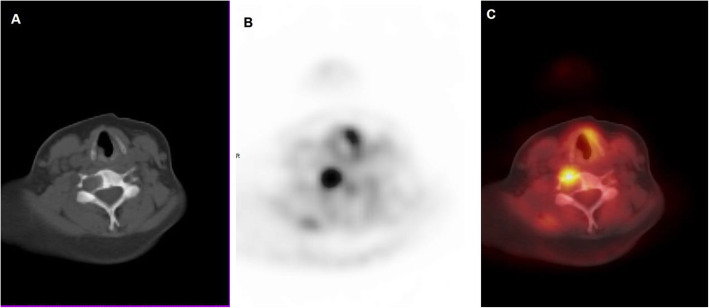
^18^F-FDG PET/CT: A axial computed tomography image showed a lytic lesion in the right side of C6 vertebral body, B axial PET images, and C fused PET/CT axial image showed FDG-avid lytic lesion in the right side of C6 vertebral body
